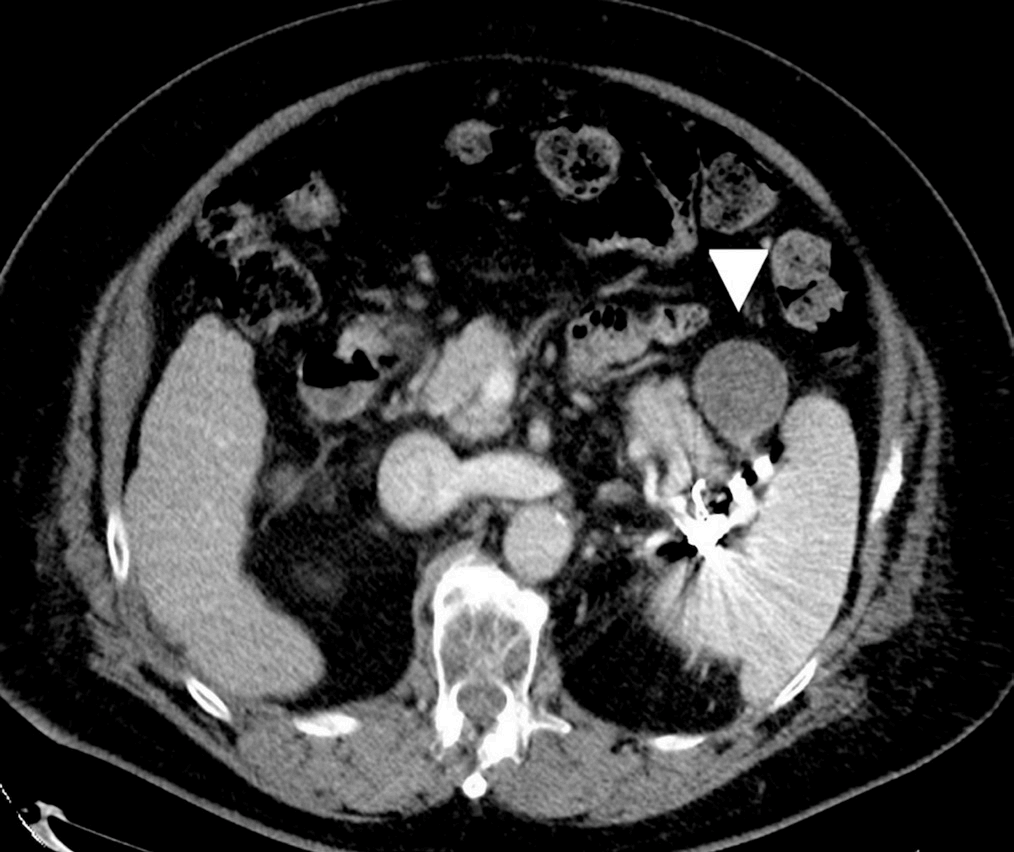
Contrast-enhanced CT image of the portal phase (10 months after B-RTO). Axial image shows the thrombosis and shrinkage of the venous aneurysm (*white arrowhead*). B-RTO, balloon-occludedretrograde transvenous obliteration
